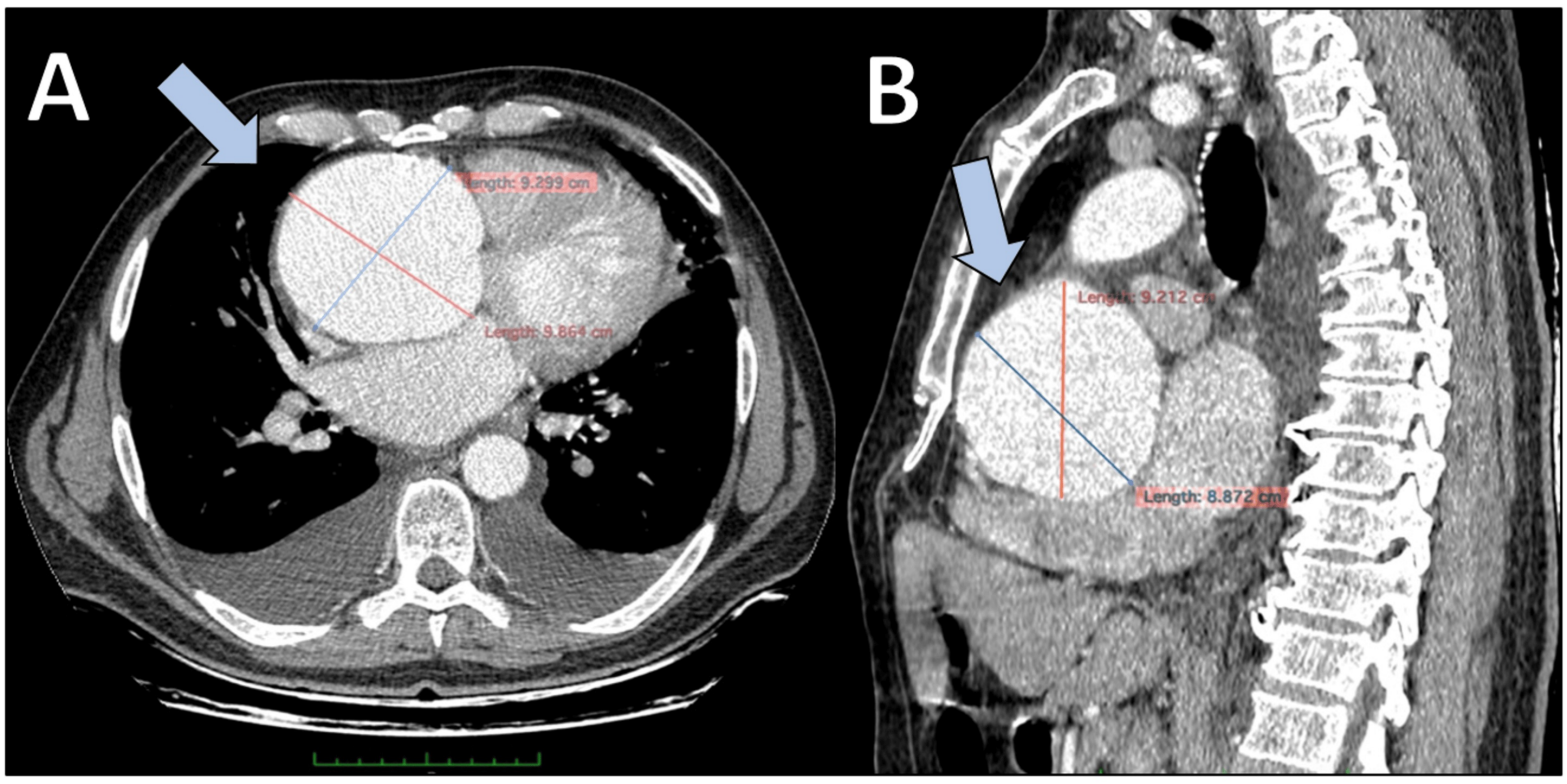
Preoperative CT images of the giant aortic root aneurysm. (A) Axial cross-sectional view demonstrating a massive aneurysmal dilation of the aortic root, measuring over 10 cm in diameter, compressing adjacent mediastinal structures. (B) Sagittal reconstruction highlighting the full extent of the aortic root dilation, with preserved continuity of the ascending aorta and absence of dissection or rupture.
